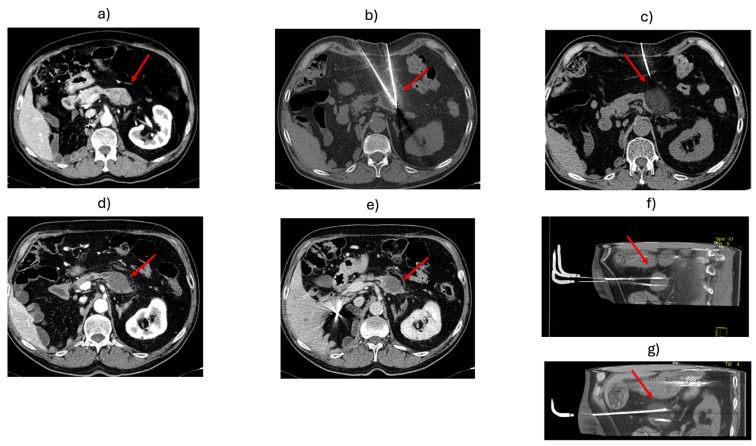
Representative case of CT-guided percutaneous cryoablation for pancreatic body adenocarcinoma. Representative case of painful unresectable pancreatic body adenocarcinoma treated with percutaneous CT-guided cryoablation. (a) Pre-procedural contrast-enhanced CT showing the pancreatic body lesion. (b,c) Axial intraprocedural CT images showing positioning of the cryoprobes within the target lesion. (d) Contrast-enhanced CT at 1-month follow-up. (e) Contrast-enhanced CT at 4-month follow-up. (f,g) Sagittal intraprocedural CT images showing positioning of the two cryoprobes along the longitudinal axis of the lesion. Arrows indicate the pancreatic lesion before treatment, the procedural target/cryoprobe position during cryoablation, and the corresponding treated area during follow-up. Follow-up imaging demonstrates persistent reduction in the contrast-enhancing component at the treated site after cryoablation.
